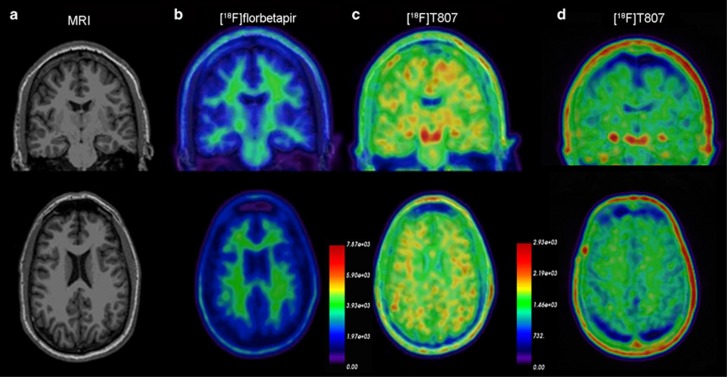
PET Imaging from a 39-year-old retired NFL player. (a) structural MRI image, (b) [^18^F]florbetapir PET (c) [^18^F]T807/AV1451 PET, (d) [^18^F]T807/AV1451 PET from a healthy age-matched control subject. Note that the [^18^F]florbetapir image was negative for amyloid accumulation, while the [^18^F]T807/AV1451 image shows extensive cortical ligand retention, especially at the junction of the gray and white matter, as is characteristic of the distribution of tauopathy in CTE. The PET scales represent ligand uptake in Bq/ml. CTE, chronic traumatic encephalopathy; MRI, magnetic resonance imaging; PET, positron emission tomography.
